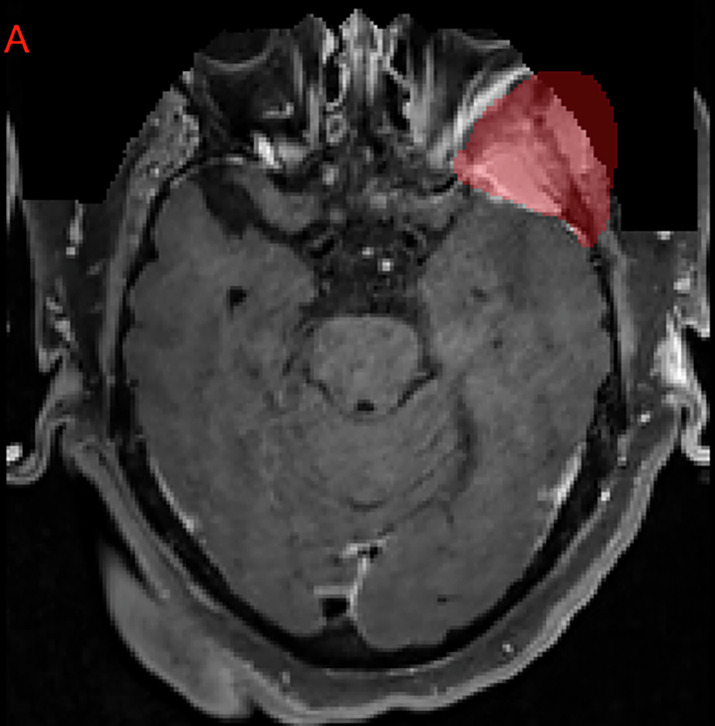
Example of an axial MRI image-label pair slice where the treating institution’s meningioma GTV extended outside of the defaced image. Note that this left sphenoid meningioma involves the skull base and extents extracranially into the left masticator space. This case was ultimately excluded from the challenge dataset due to the excessive volume within the anonymized region that would have needed to be reintroduced.
